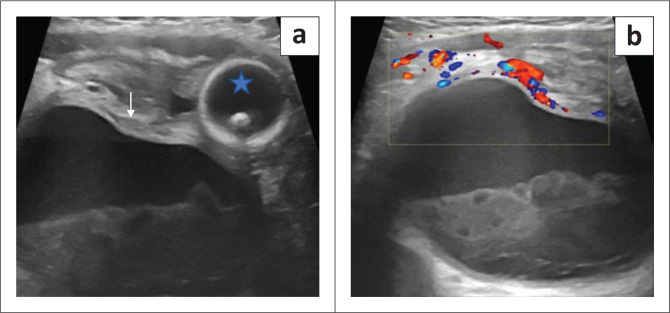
(a) Transverse grey scale and (b) colour doppler ultrasound images demonstrated a well-defined, anechoic, cystic lesion (*white arrow*) with dependent debris. The urinary bladder with a foley’s catheter bulb (star) is seen separately.
